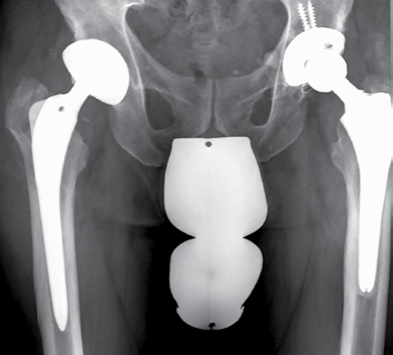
Preoperative x-ray of a 65-year old male patient 5 and 12 years after primary arthroplasty on the left, respectively right side. There are osteolytic bony changes in the region of the greater trochanter.
